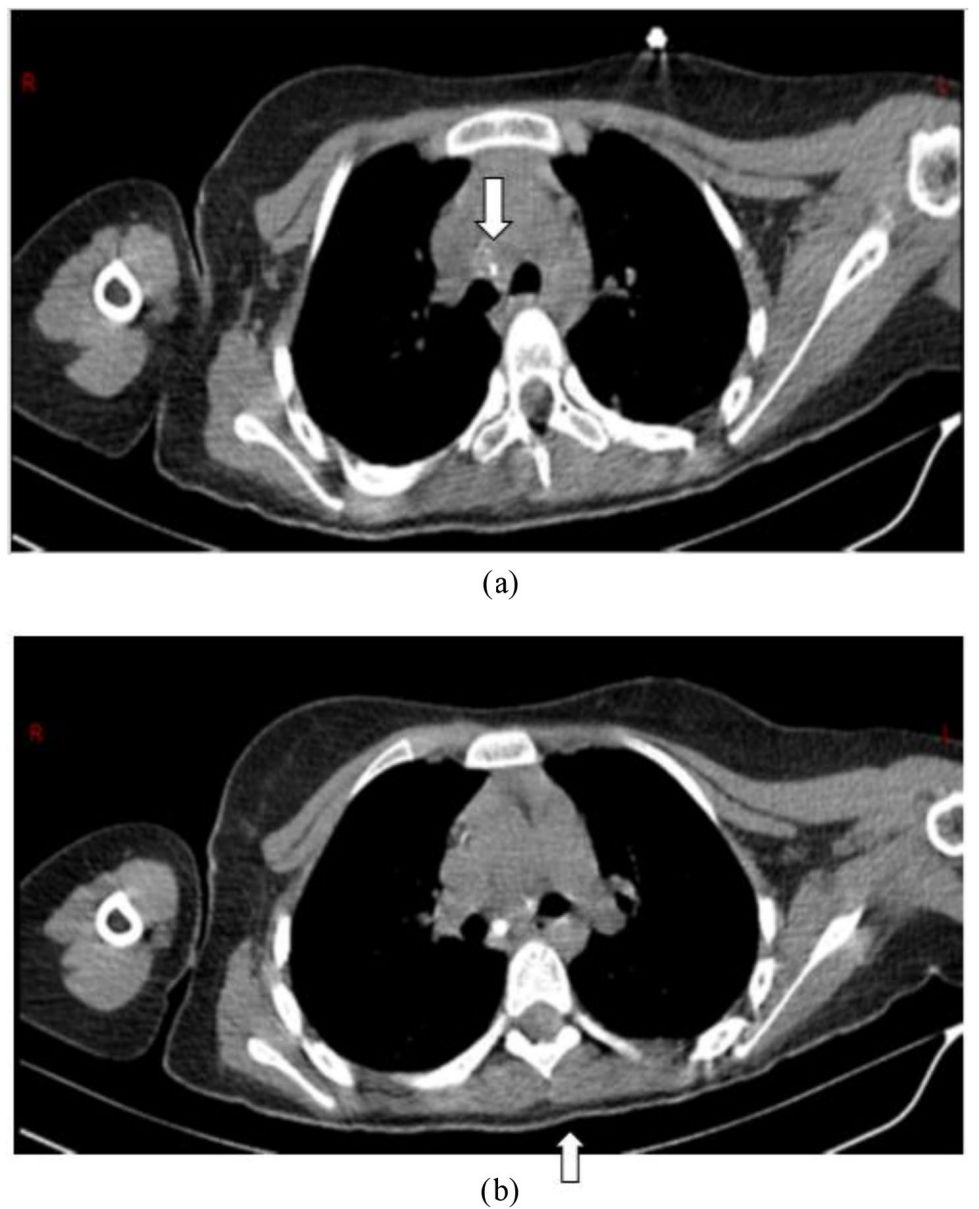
a, b) Chest CT scan without contrast (mediastinal window): Some calcified lymphadenopathies in the right paratracheal and subcarinal space of the middle mediastinum (white arrows)
